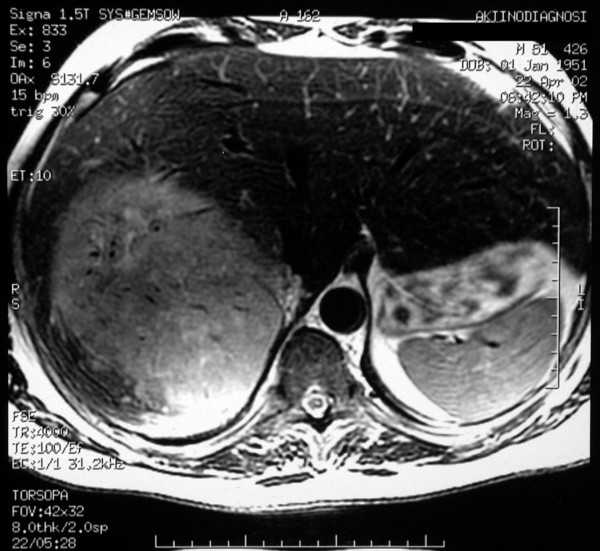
Abdominal MRI showing the hepatic invasion, which was submitted to en block resection with the right adrenal.
